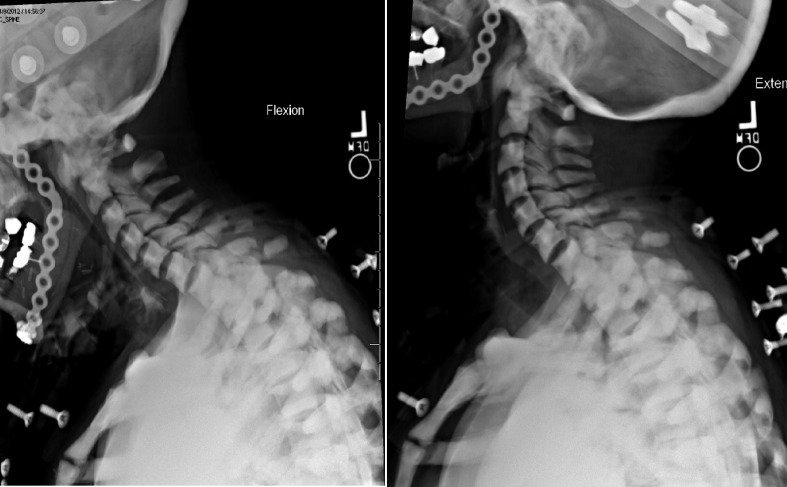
Cervical spine flexion and extension radiographs.
